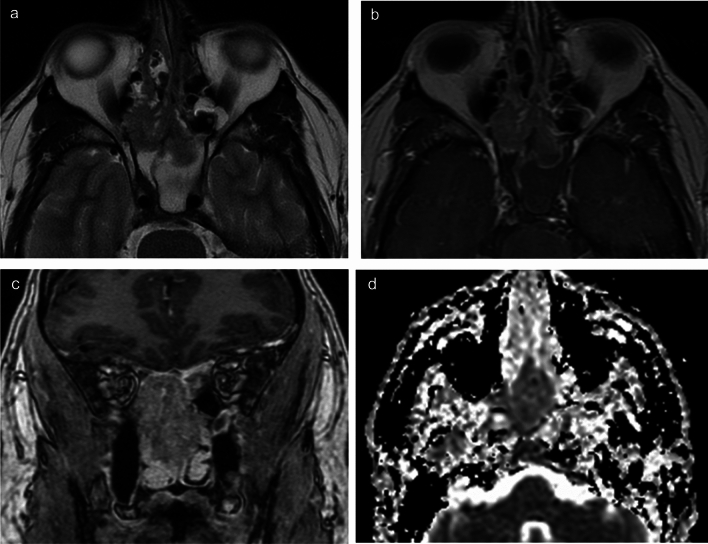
Sinonasal nuclear protein in testis carcinoma (case 2). T2-weighted axial image (a) shows a tumor with slightly high signal intensity extending from the right ethmoid sinus to the bilateral sphenoid sinuses. Post-contrast T1 axial image (b) and coronal images (c) show heterogeneous slight enhancement. The lesion is confined to the paranasal sinuses with no surrounding invasive or destructive changes, no intracranial extension, and no intraorbital extension. ADC map (d) demonstrates a value of 1.08 × 10⁻^3^ mm^2^/s within the lesion
